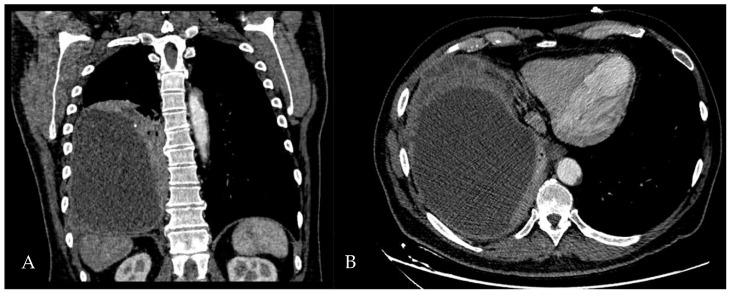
Chest CT scan of the mediastinal window in the coronal (A) and axial (B) plane, showing fluid collection in the medium–lower parts of the right hemithorax (maximum axial dimeters of 19 × 13 cm; craniocaudal extension of 16 cm) with thickened walls and contrast enhancement. There is also a complete atelectasis of middle and lower lobes and partial atelectasis of the upper lobe.
